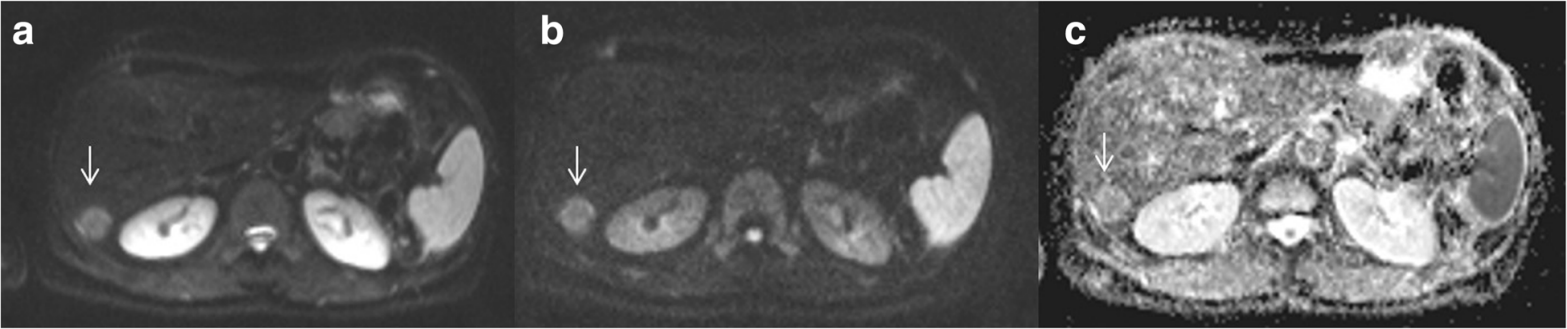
Low-grade HCC. DWI sequences: in A b50 s/mm^2^; in B b800 s/mm2 and in C ADC map
